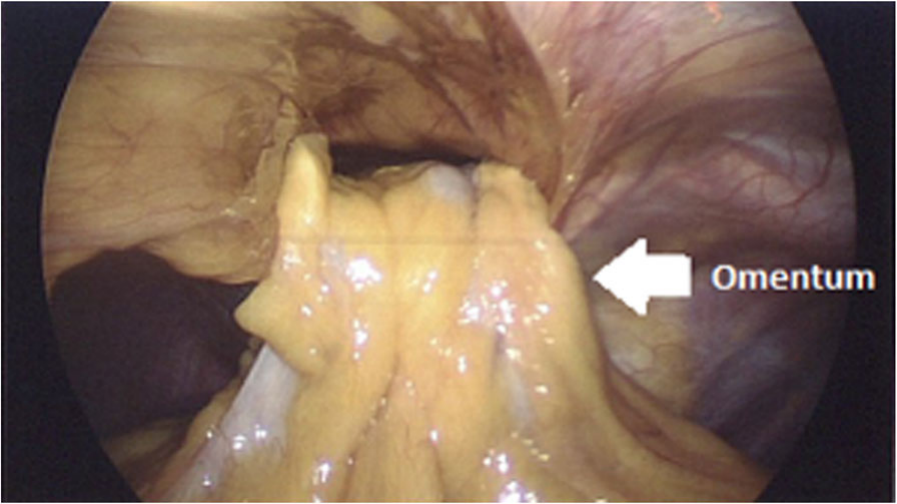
Computed tomographic scan (sagittal section) with *arrow* showing the diaphragmatic defect and herniated bowel loops in the pleural cavity
